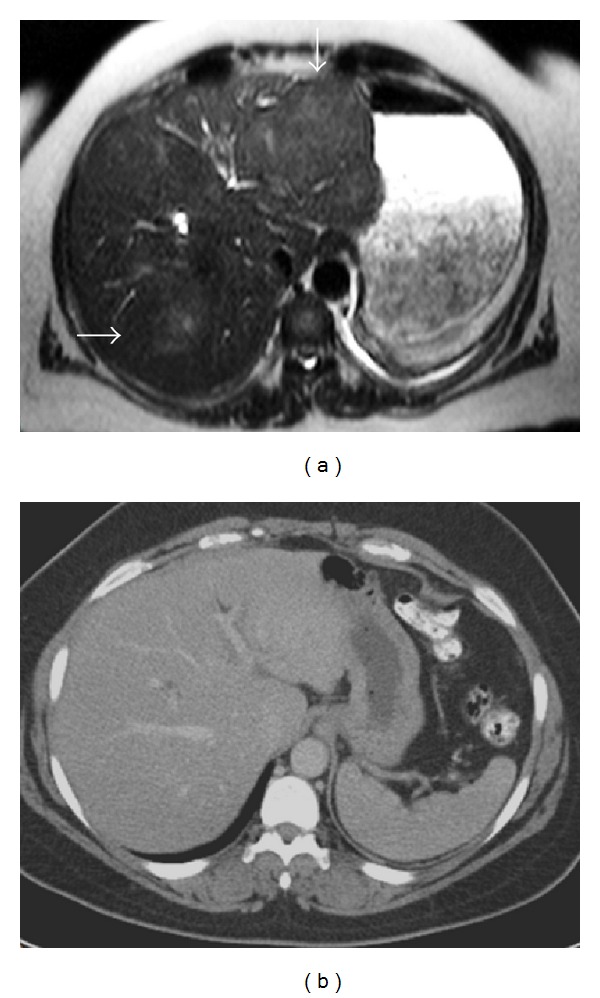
Radiologic appearance of IMT lesions in the liver before (a) and after treatment (b) with ALK-inhibitor. (a) MRI of the abdomen with and without Gadolinium before treatment demonstrates vague poorly defined liver masses in the right and left lobes (arrows) each measuring approximately 6-7 cm. (b) CT of the abdomen obtained at 15-month follow-up demonstrates resolution of the ill-defined liver masses bilaterally following treatment with ALK-inhibitor.
